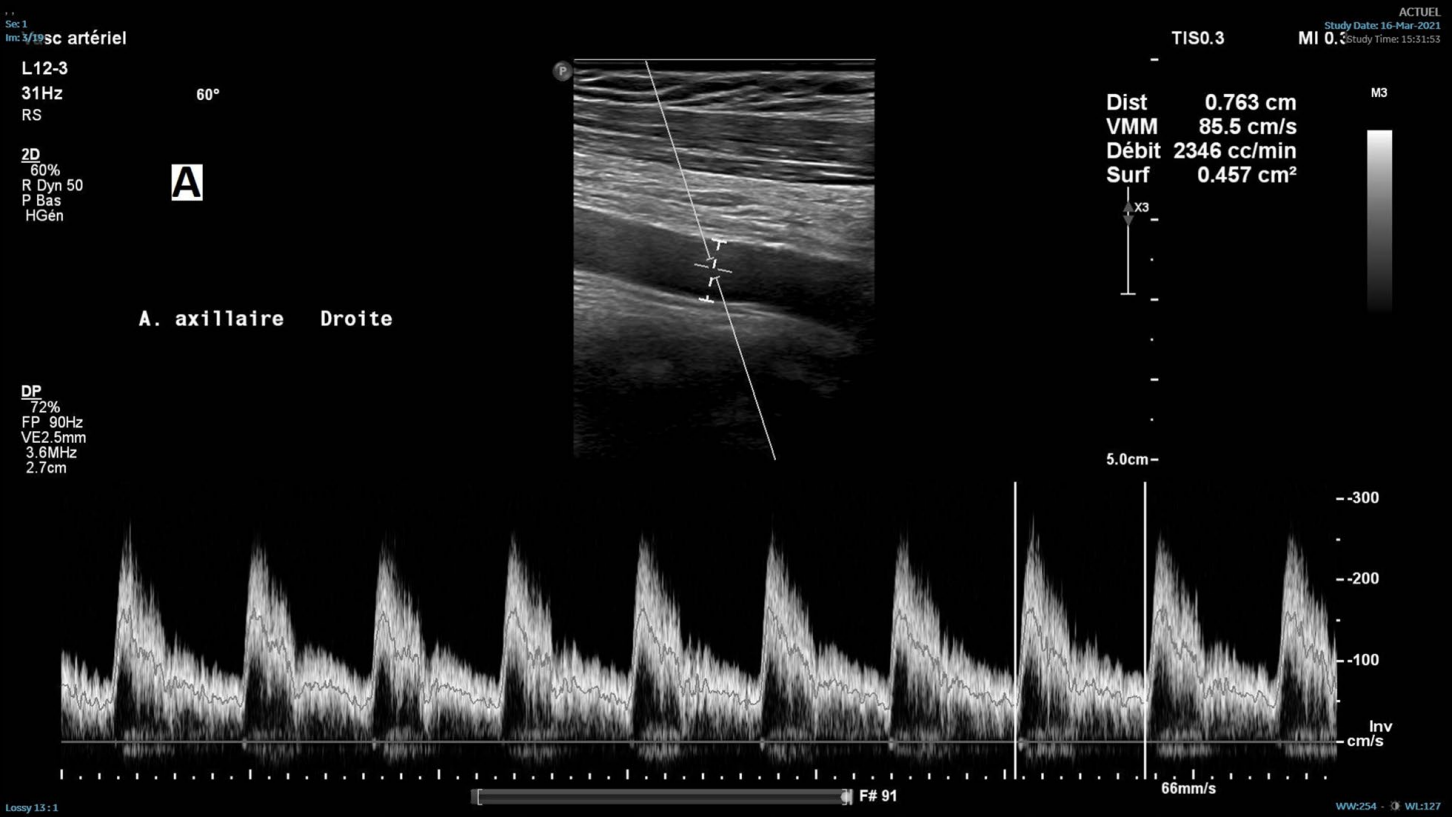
Systolo-diastolic Doppler velocity waveform in the right axillary artery with estimated flow of 2.35 L/min
